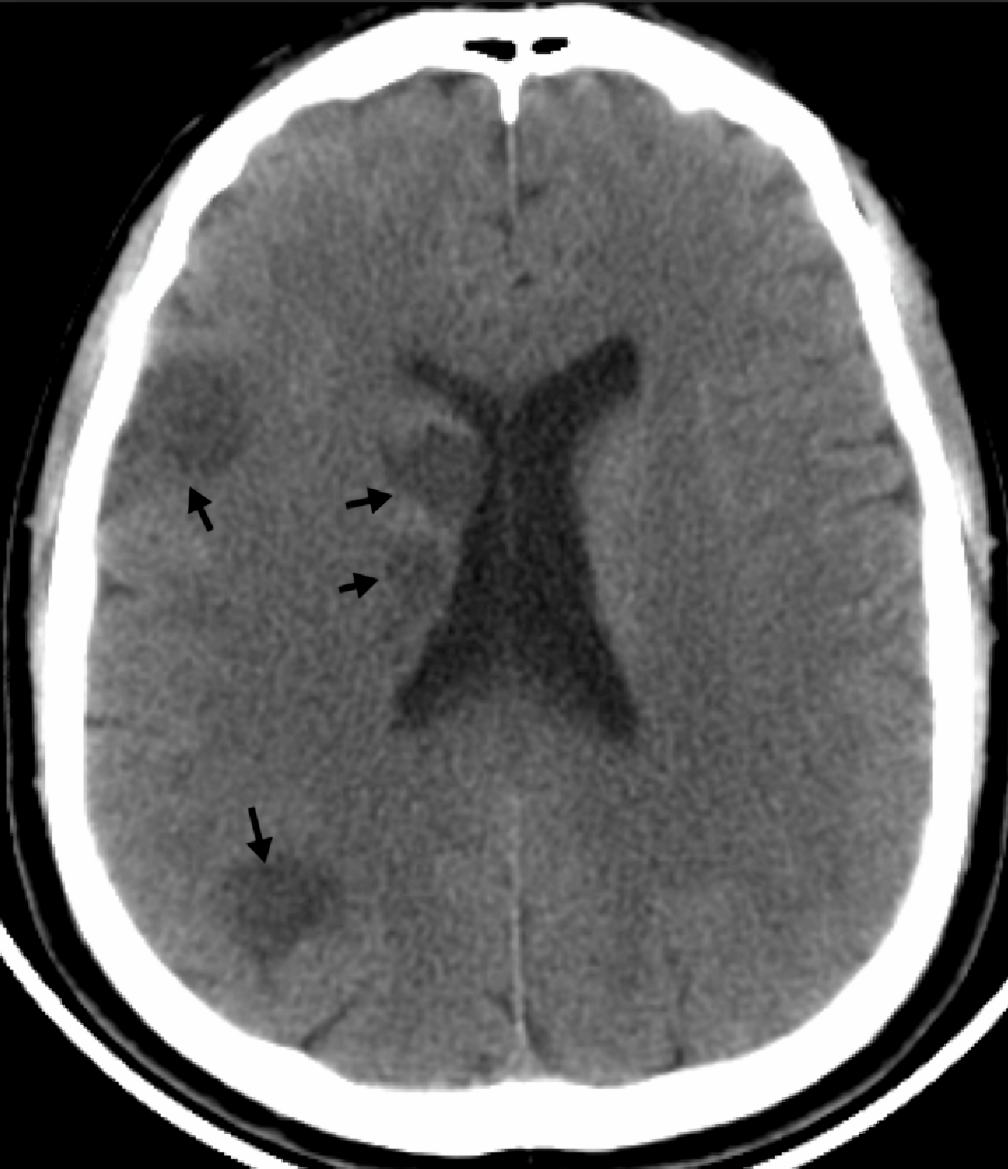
Non-enhanced computed tomography (CT) scan showed multiple subacute ischemic lesions (black arrows) within the right middle cerebral artery (MCA) territory.
